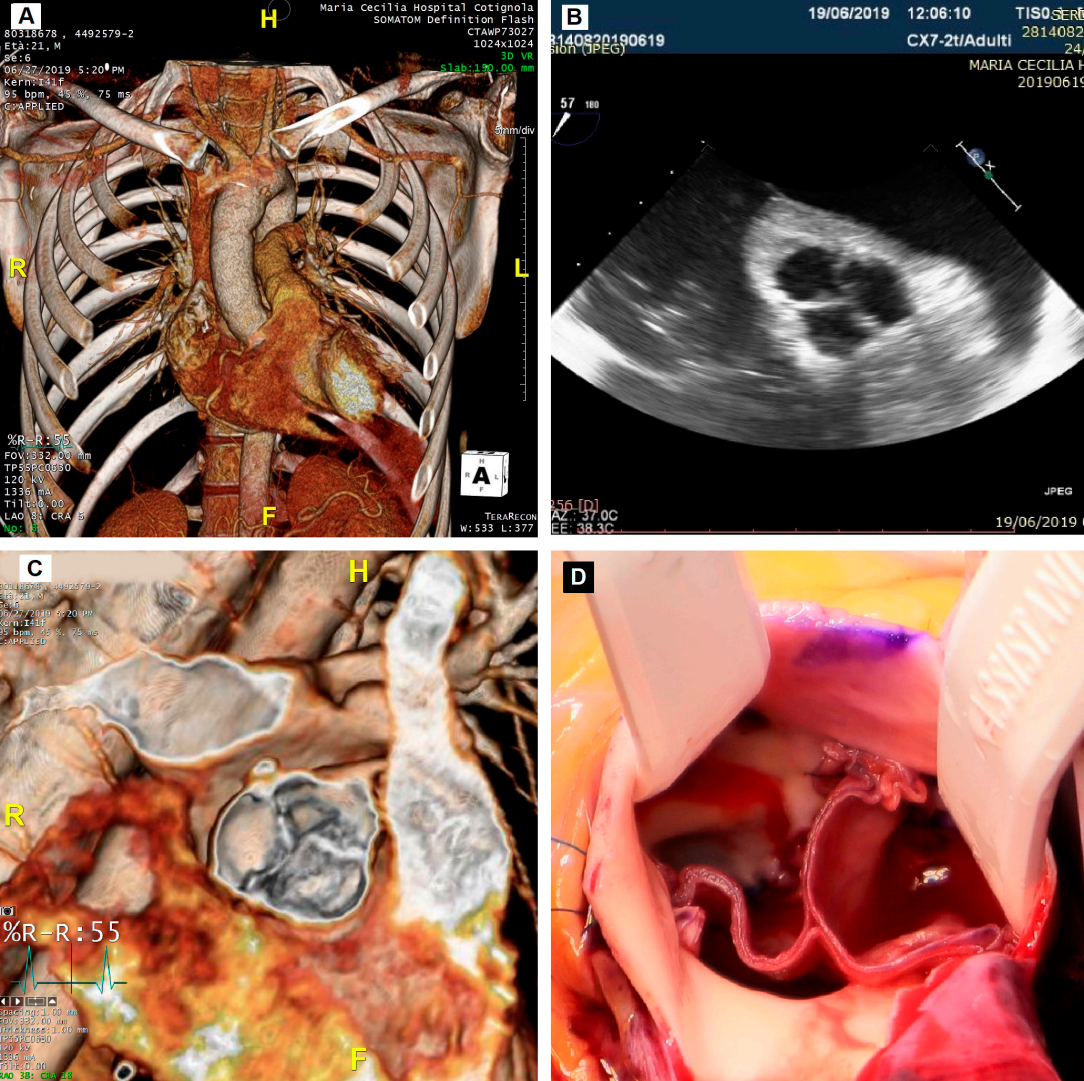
Post- and intra-operative imaging. (A) Chest CT scan with 3D reconstruction - General view of the heart and the aorta after repair. (B) Postoperative trans-oesophageal echocardiogram. (C) Chest CT scan with 3D reconstruction - Planar view of the neo-tailored tricuspid aortic valve. (D) Surgical view of the neo-tailored tricuspid aortic valve.
